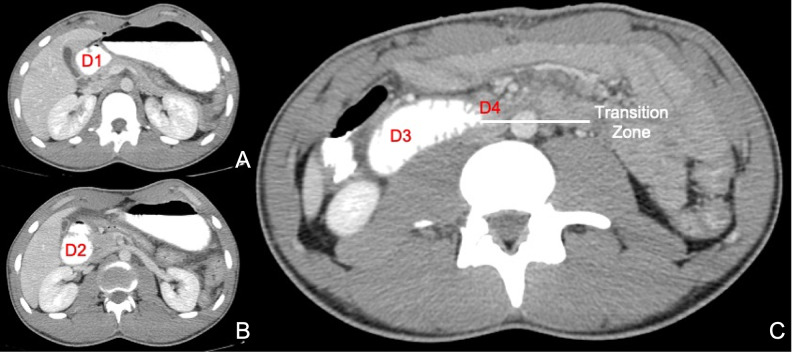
Postoperative CECT Axial views showing pooling of contrast up to D2 (A, B) and compression of D3 with a transition zone between D3 and D4 (C) D1: first part of duodenum; D2: second part of duodenum; D3: third part of duodenum; D4: fourth part of duodenum; CECT: contrast-enhanced computed tomography
